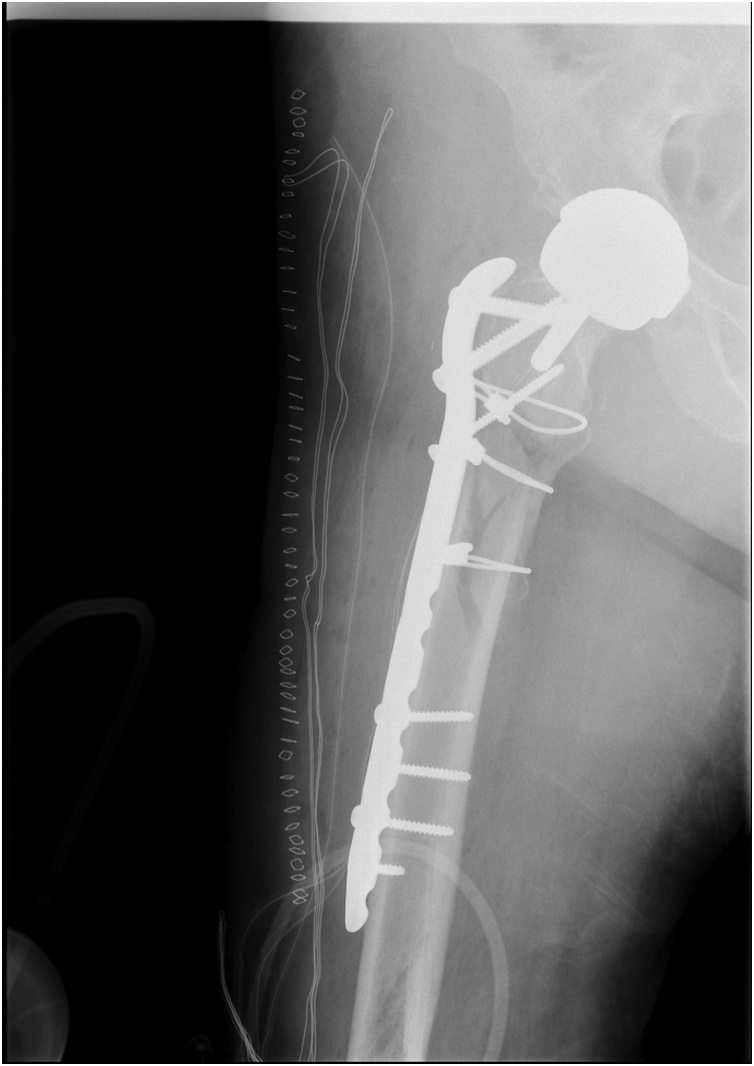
Postoperative radiograph. Internal fixation with trochanteric plate and cerclages.
